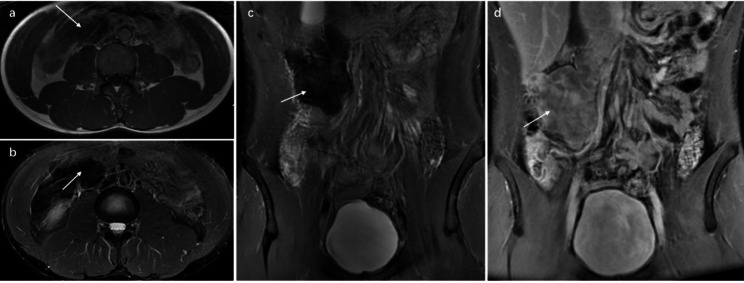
MRI image of RNFP. The irregular masses (white arrow) showed moderate hypointensity on axial T1-weighted MR images (a), and significant hypointensity on axial and coronal T2-weighted MR images (b, c). They exhibited progressively mild enhancement, with minimal enhancement in the arterial phase and gradually increased enhancement in the portal venous and delayed phases on postcontrast MRI (d)
